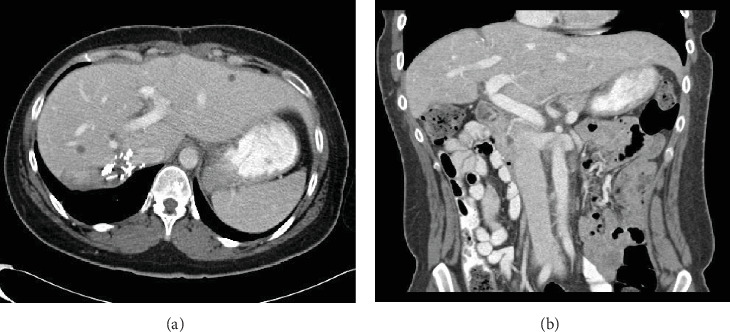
Computed tomography of the abdomen 6 months following giant hemangioma resection. (a) Axial image showing normal residual liver with surgical clips along the edge of inferior vena cava (IVC). (b) Coronal imaging showing resolution of prior displacement of abdominal viscera and IVC.
